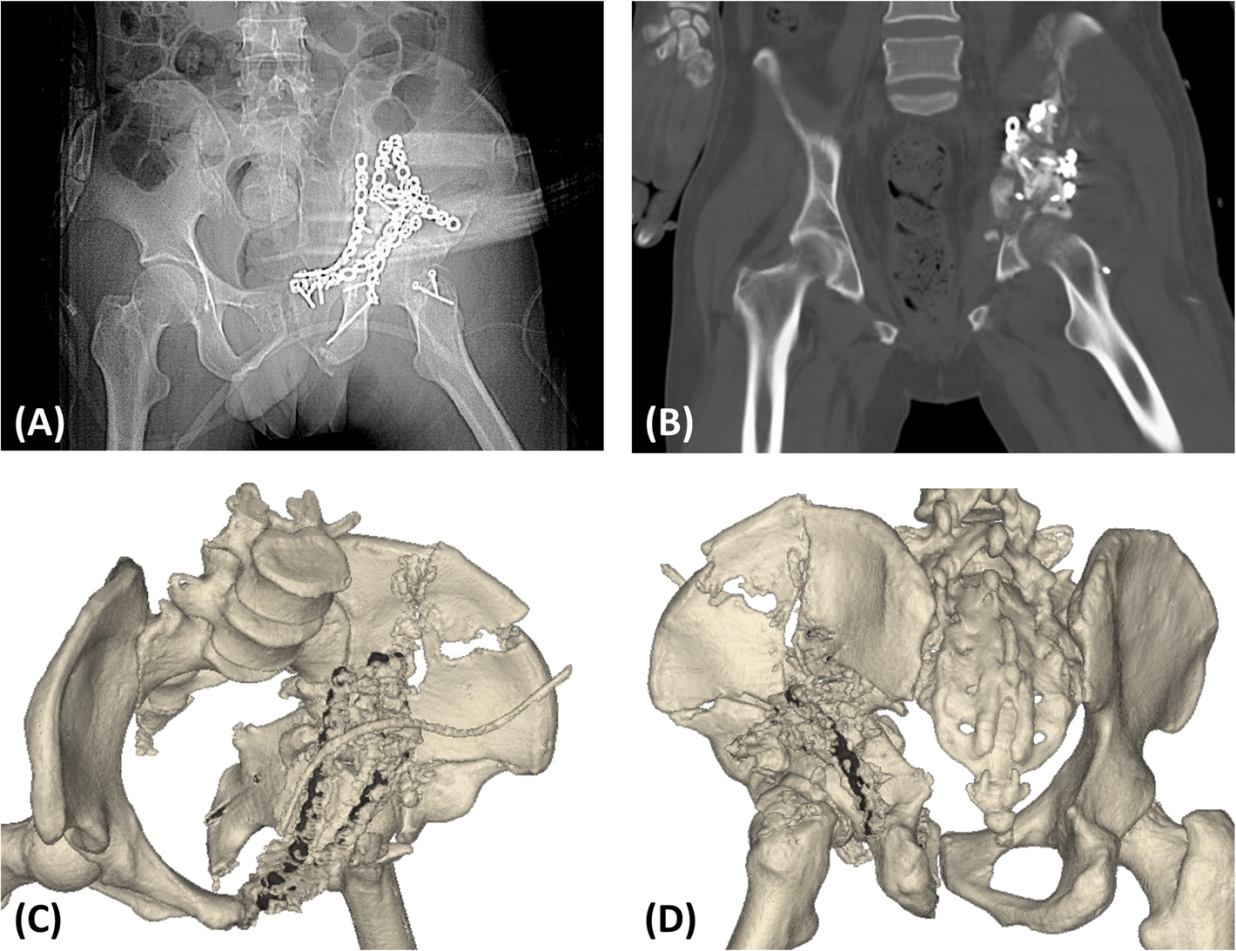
a The anteroposterior radiographs, b computed tomography scan, and (c and d) three-dimensional reconstruction of the postoperative pelvis were performed. The accuracy of reduction was imperfect. The orientation and length of all screws were suitable, and no articular penetration occurred
